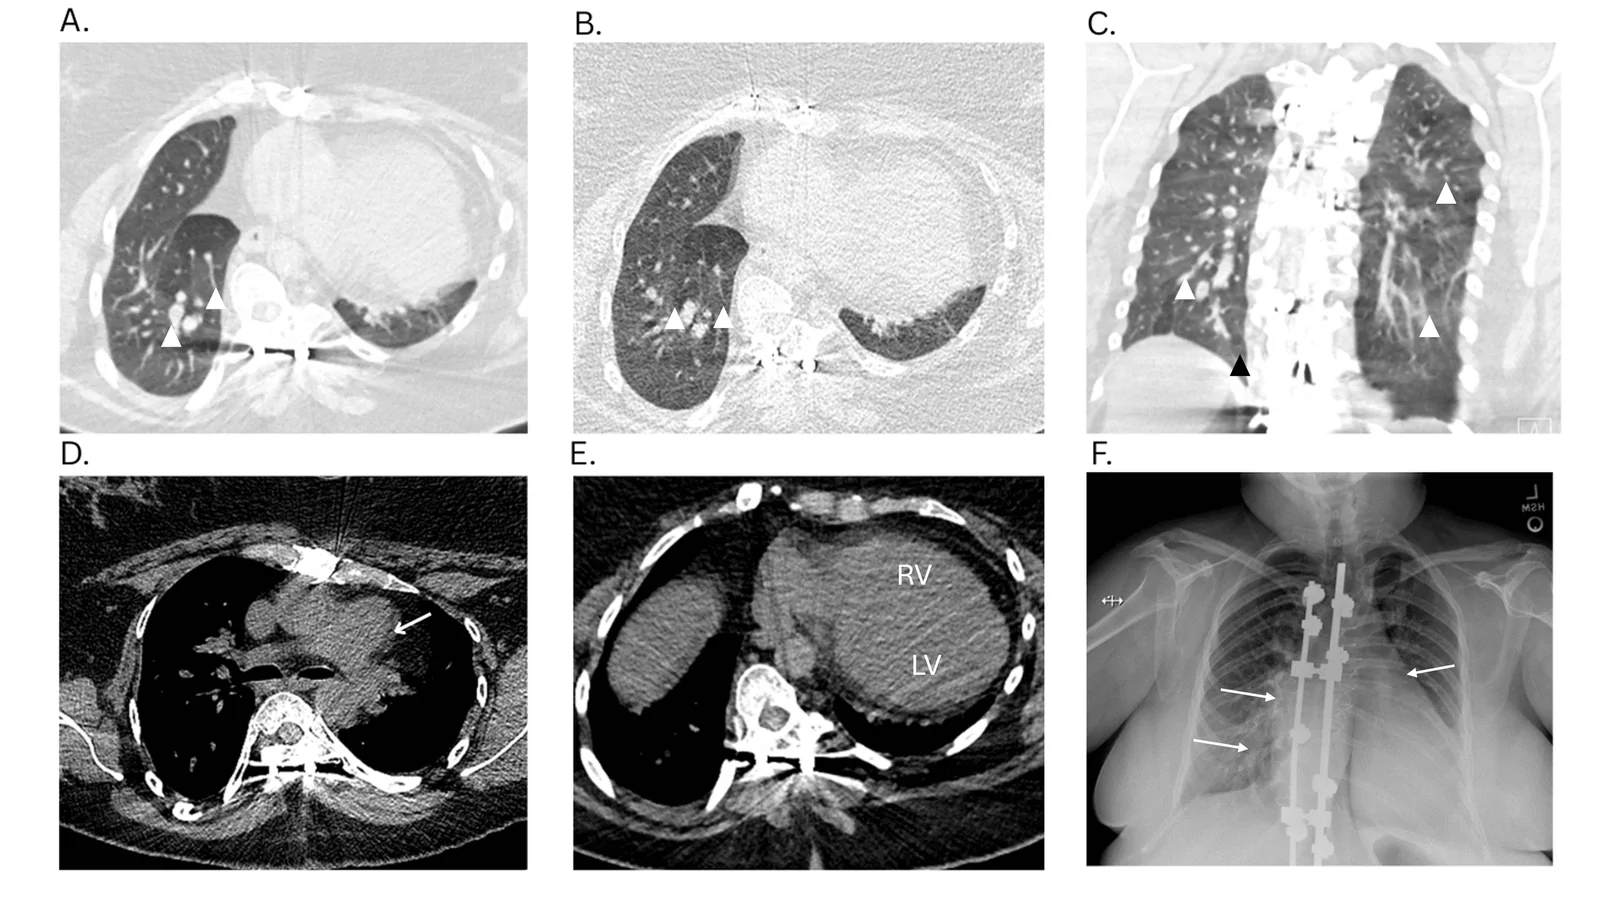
Imaging findings in Case 4. Axial and coronal computed tomography (CT) images show mild mosaic attenuation (arrowheads) with relative attenuation of the peripheral pulmonary arteries (A-C), enlargement of the main pulmonary artery (D, arrow), and biventricular enlargement (E). The CT images are limited by artifacts from spinal fusion hardware and sternotomy wires. A pectus excavatum deformity is also present. A frontal chest radiograph (F) demonstrates a large body habitus, cardiomegaly, and enlargement of the hilar and interlobar pulmonary arteries.
